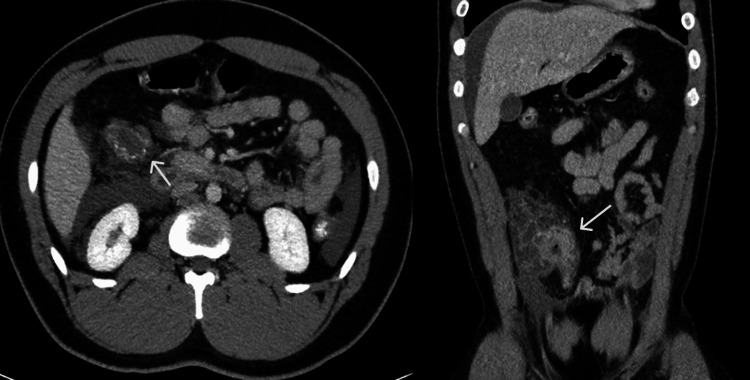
Axial and coronal CT images of the abdomen and pelvis. Long segment of cecum/ascending colon that demonstrates mural thickening, mucosal hyperenhancement, and significant adjacent fat stranding, with multiple local enlarged lymph nodes.
